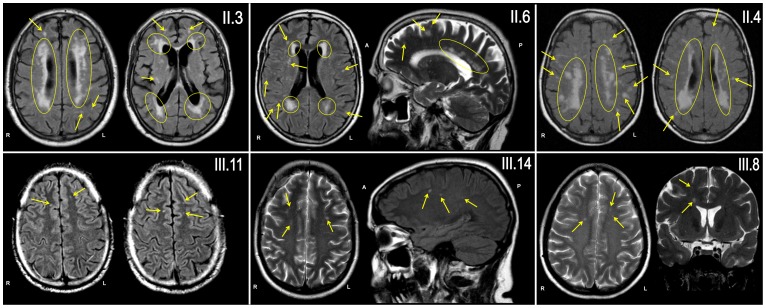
Punctuated (arrows) and confluent (yellow circles) WML were present on MR images (axial and sagittal FLAIR- and T2-sequences) of all six examined family members, all carrying D313Y. Only patient II.3 had mild cardiovascular risk factors (treated arterial hypertension). Extent and lesion load were age-related, but WML were already present in young family members without any vascular risk factor (patient III.8, III.11 and III.14; 49, 34 and 41 years of age, respectively).
